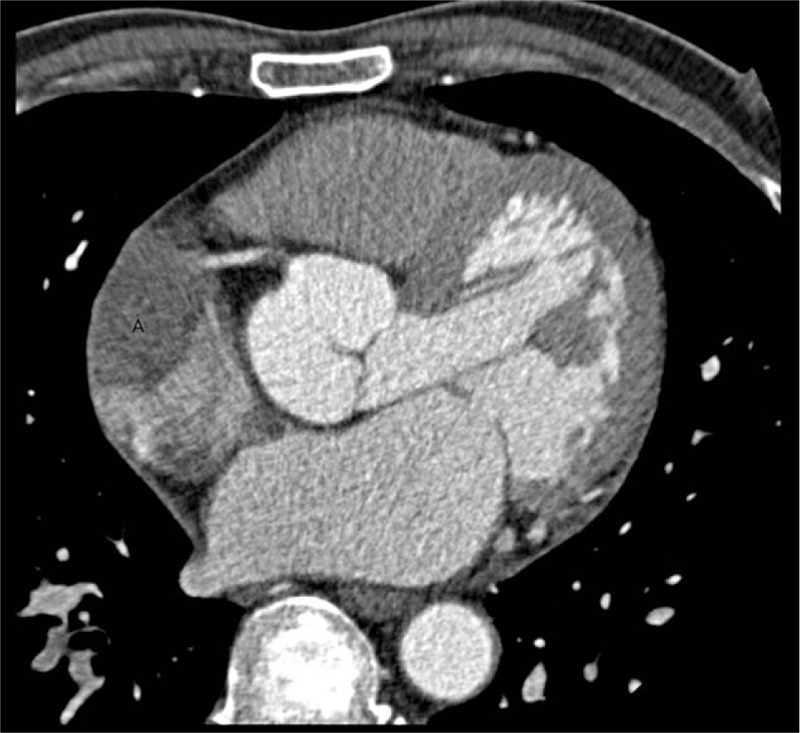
Computed tomography showing a 3.5-cm filling defect at the right atrium of the heart.
